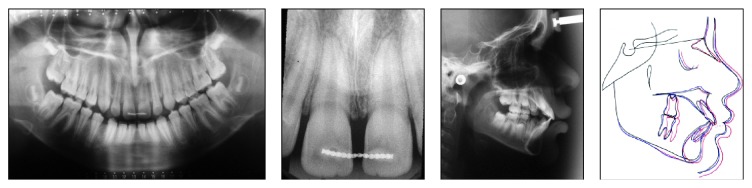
Posttreatment radiographs and cephalometric measurements.
